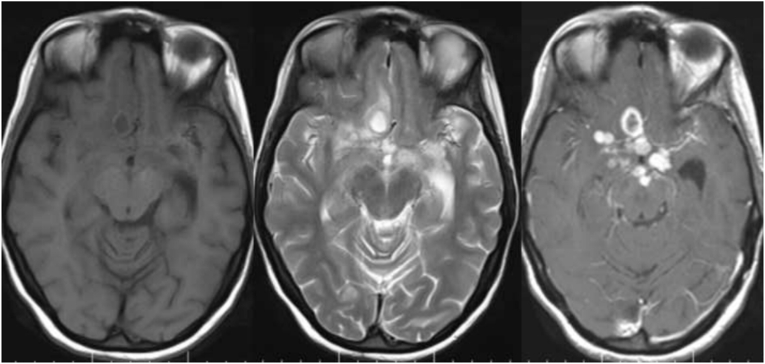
noncaseating granuloma. (A) tuberculoma in the frontal and suprasellar regions, hypointense in the T1-weighted axial image, (B) hyperintense in the T2-weighted axial image, and (C) ring contrast enhancement of the tuberculoma on the post-contrast image.
